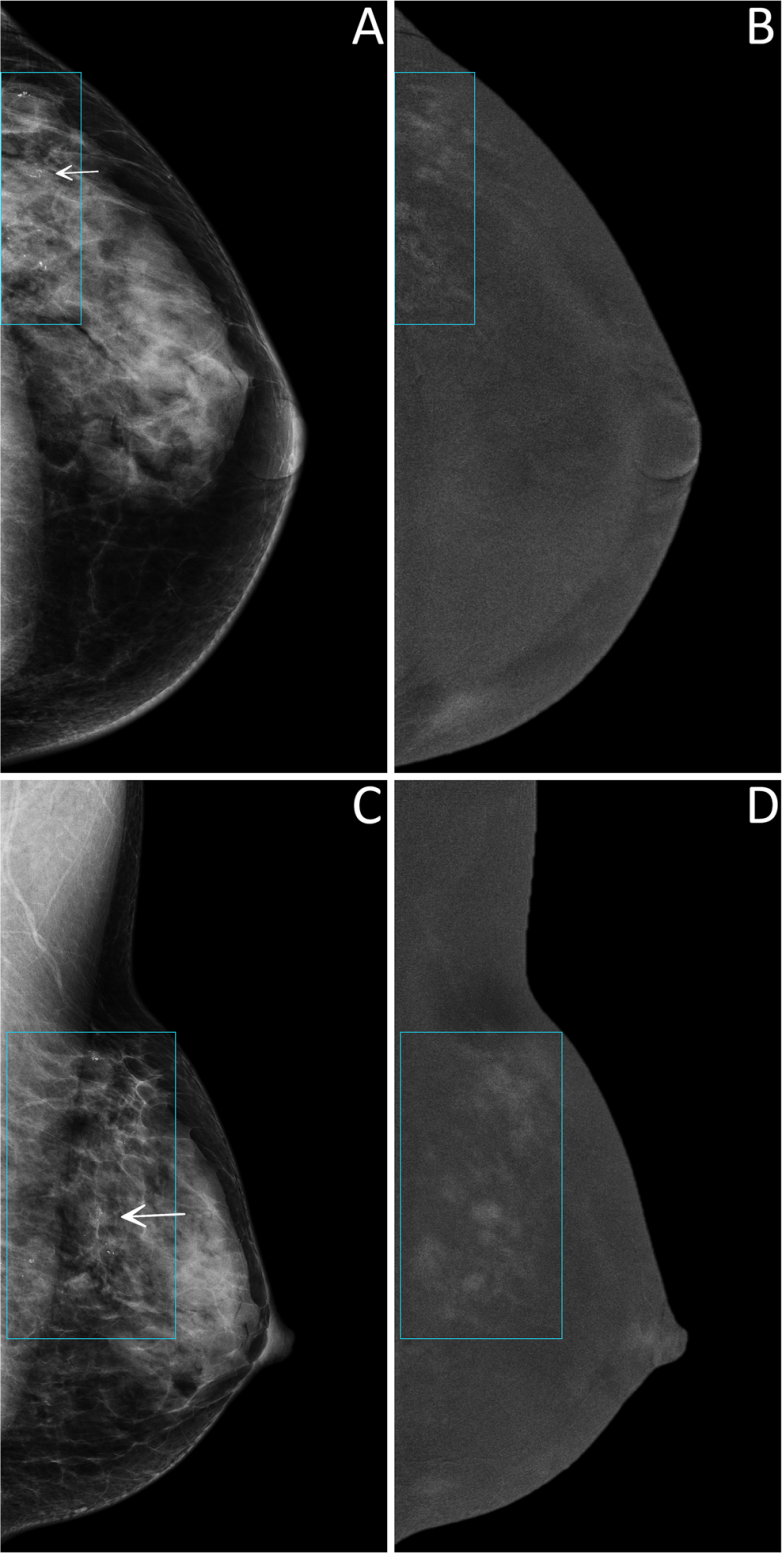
True positive case at contrast-enhanced mammography. A 53-year-old woman was recalled for suspicious calcifications in the left breast. An ultrasound-guided core needle biopsy was performed, resulting in a diagnosis of grade 2 ductal carcinoma in situ. Low-energy images (panels A and C) show multiple groups of pleomorphic calcifications in the left upper-outer quadrant (white arrows in light blue rectangles). Recombined images (panels B and D, light blue rectangles) revealed an area of non-mass enhancement involving the whole upper-outer quadrant
